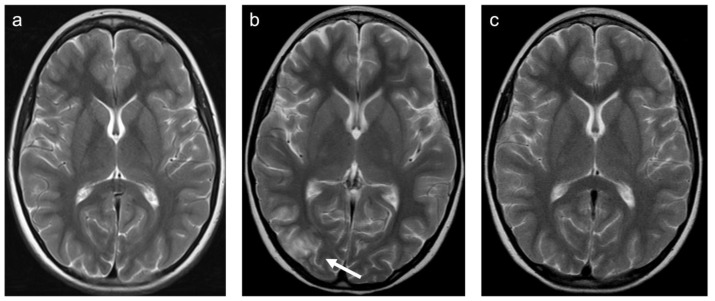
Representative case “Toxic white matter injuries”: L-asparaginase treatment. T2W (a–c) sequences. ten-year-old boy with induction of L-asparaginase treatment of acute lymphoblastic lymphoblastic leukemia. MRI prior to treatment (a), shortly after induction of treatment showing vasogenic edema within the right (white arrow) and, of lesser extent, left occipital region ((b); clinical presentation: epileptic seizure) with patchy enhancement in a leptomeningeal pattern (not shown) and restitution after treatment stop (c).
